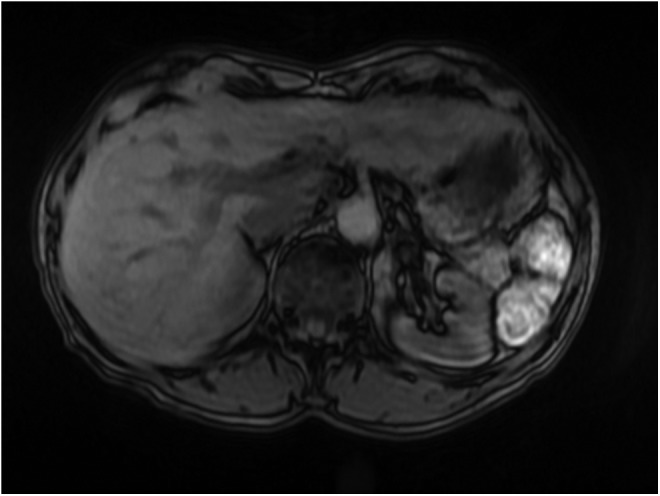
MRI of the abdomen showing normal appearance of the left renal pelvis in which high-grade transitional cell tumor was discovered on ureteroscopy.
